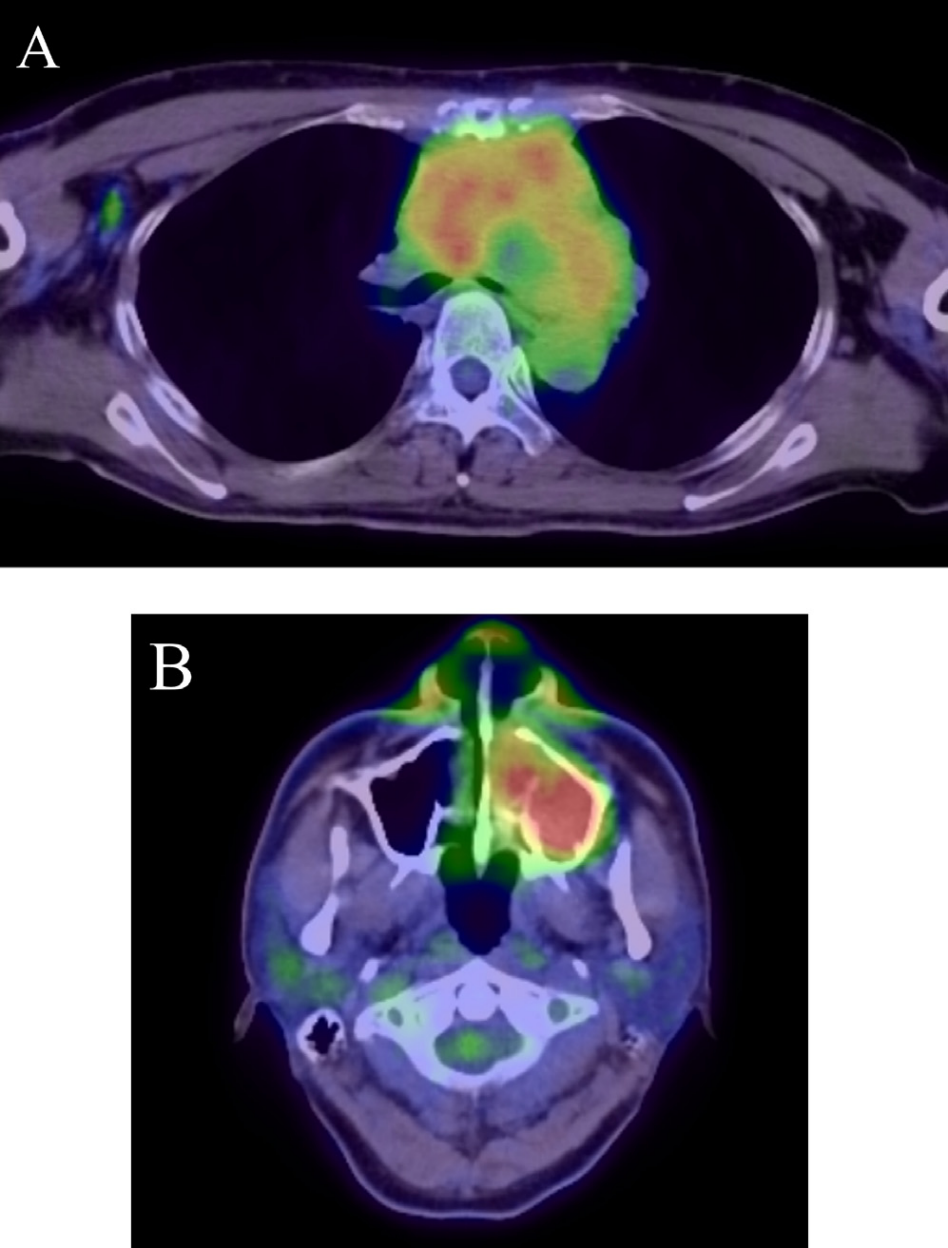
FDG PET-CT scan of chest(A), paranasal sinuses(B) in the second case. FDG PET-CT scan showing abnormal uptake in the mediastinum and right axillary lymph nodes (SUVmax = 2.0-7.5) (A) and in the left maxillary sinus (SUVmax = 8.0)(B).
